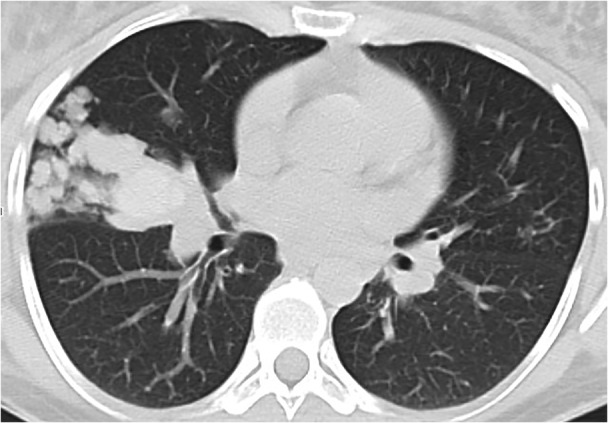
Middle lobe consolidation with polylobulated morphology on chest computed tomography
